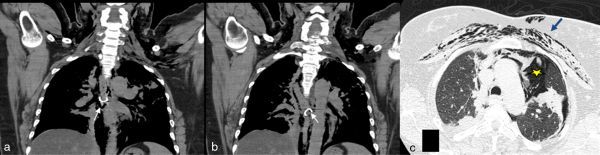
(a, b) Plain CT scan of the chest in coronal section showing a metallic density foreign body (white arrows) in the middle one-third of the oesophagus. (c) Axial non-contrast-enhanced CT scan showing pneumomediastinum (yellow star), subcutaneous emphysema (blue arrow) and left pneumothorax.
